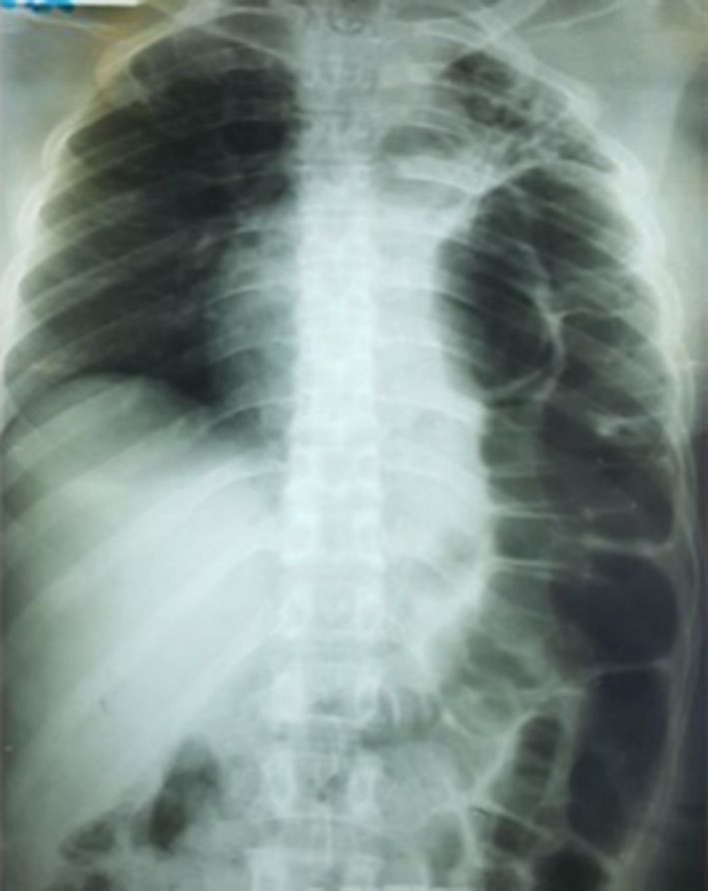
Abdominal x-rays showing left diaphragmatic hernia
